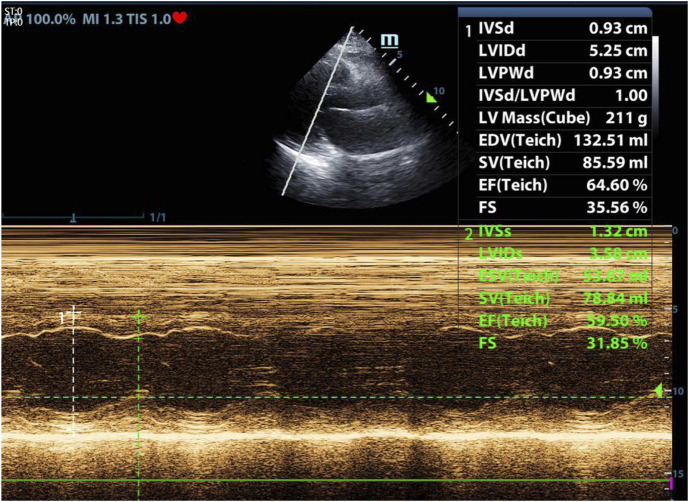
Echocardiography during SBT-T after reintubation. Left ventricular ejection fraction (LVEF): 59.5% (with permission from the patient.)
